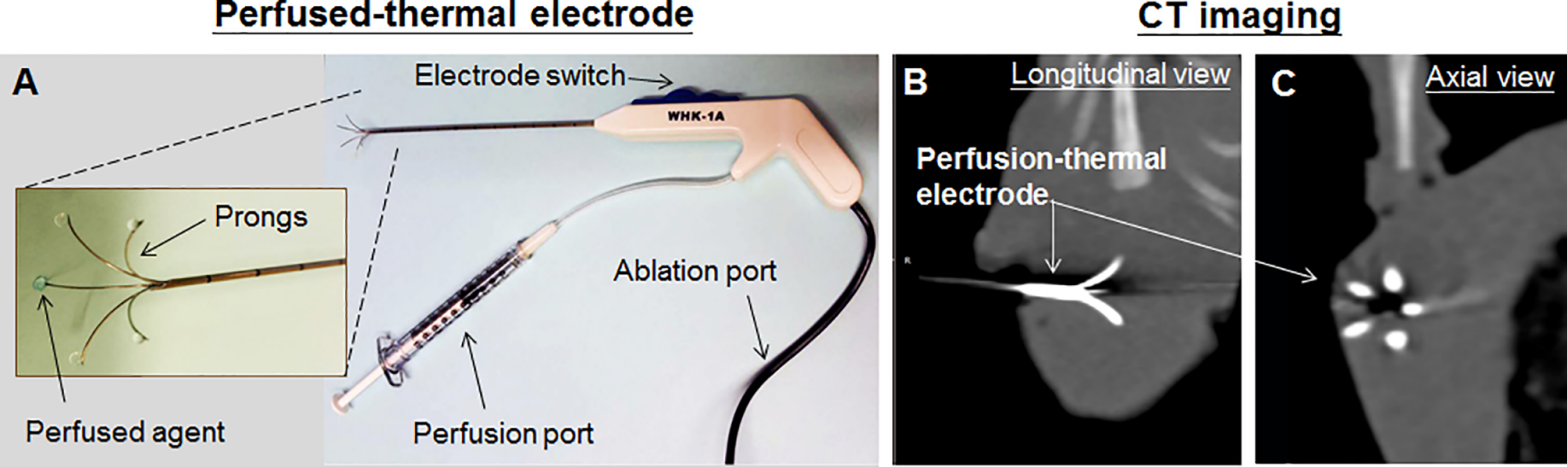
(A) The perfusion-thermal RF electrode. There is a tunnel within each of electrode prongs, which enables to perfuse agents into the ablated tumor periphery *via* the perfusion port. (B, C) The deploy size of the electrode prongs can be controlled *via* the electrode switch and precisely monitored under CT.
